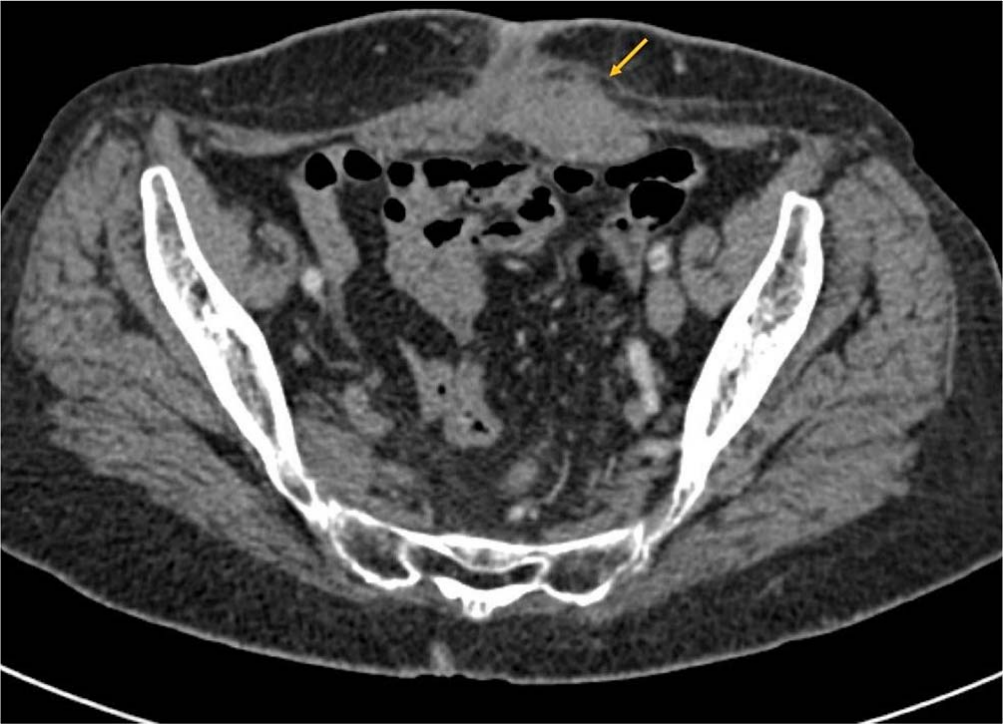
Axial CT scan showing a significant reduction in the size of the mass post-chemotherapy, now confined to the left rectus abdominis muscle with fat infiltration.
